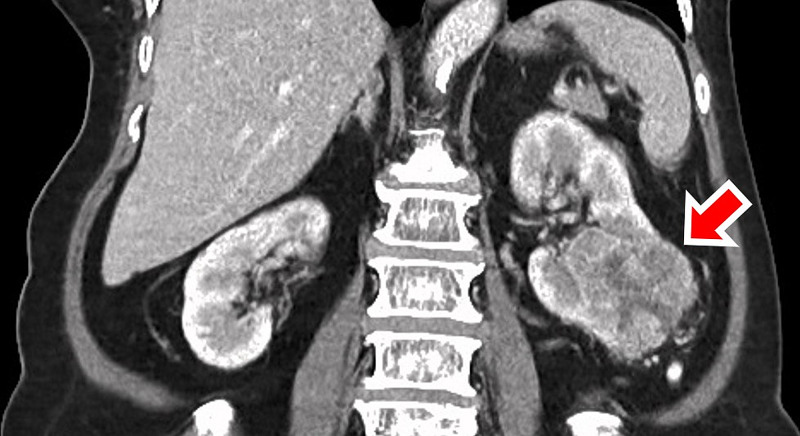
CT abdomen revealed left RCC (red arrow). RCC: Renal cell carcinoma.
